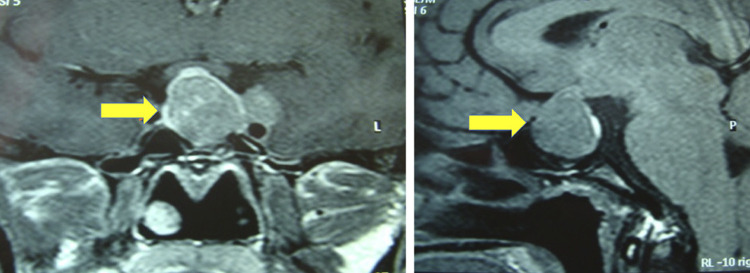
Coronal (left) and sagittal (right) magnetic resonance imaging of the pituitary in a 28-year-old female patient with a prolactin level of 343 ng/mL and a 2.8 cm macroadenoma (arrows) that was initially labeled a cabergoline-resistant prolactinoma. After polyethylene glycol (PEG) precipitation, the prolactin level decreased to 27.1 ng/mL (normal range < 29 ng/mL). The patient received a final diagnosis of gonadotroph pituitary adenoma associated with macroprolactinemia.
